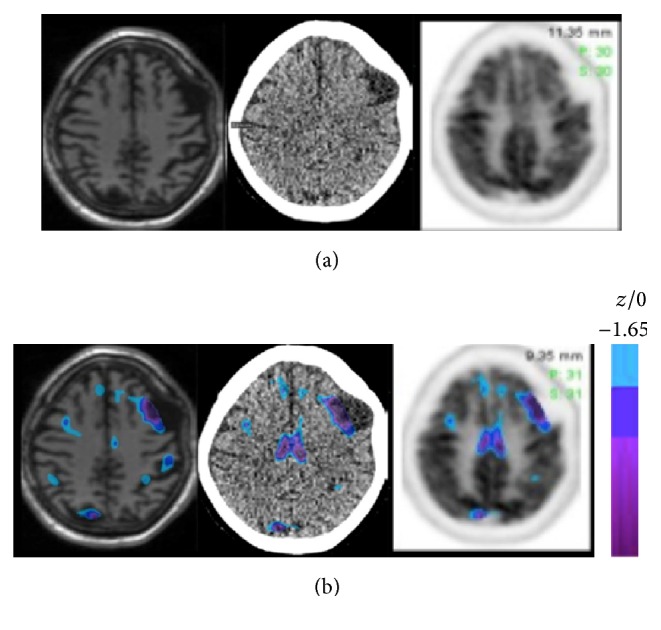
Corresponding transaxial MRI, CT, and FDG PET images (a) with superimposed FDG PET *z*-score images on (b). Findings show a left frontal extra-axial anatomic lesion compatible with arachnoid cyst and focally localized adjacent left dorsolateral frontal cortical glucose hypometabolism on FDG PET. Glucose metabolic reductions greater than 1.65 SD below the normal mean are shown in color overlay.
